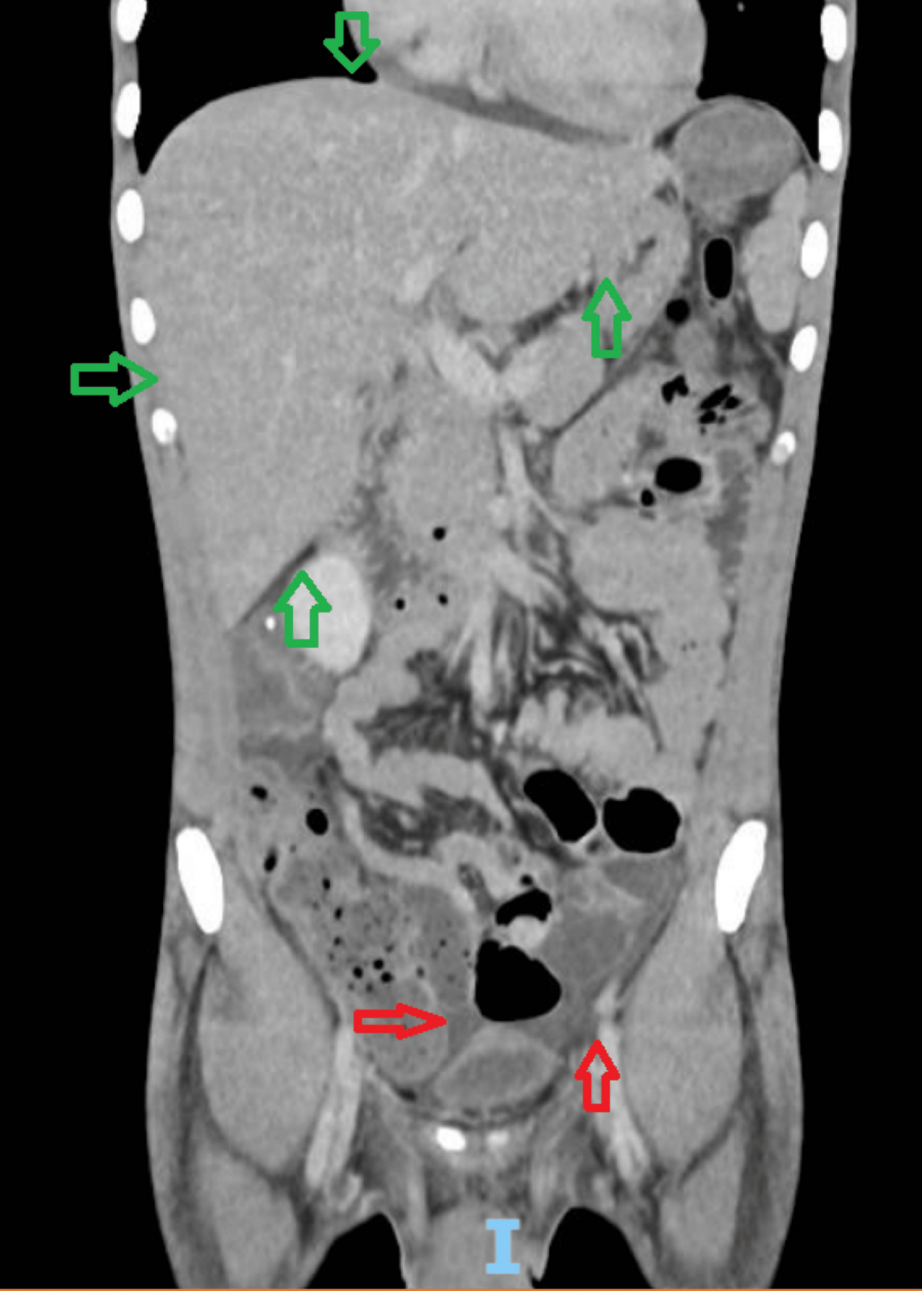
Axial contrast-enhanced abdominal CT scan demonstrating homogeneous hepatosplenomegaly (green arrows) and a small volume of ascites (red arrows) CT: computed tomography
